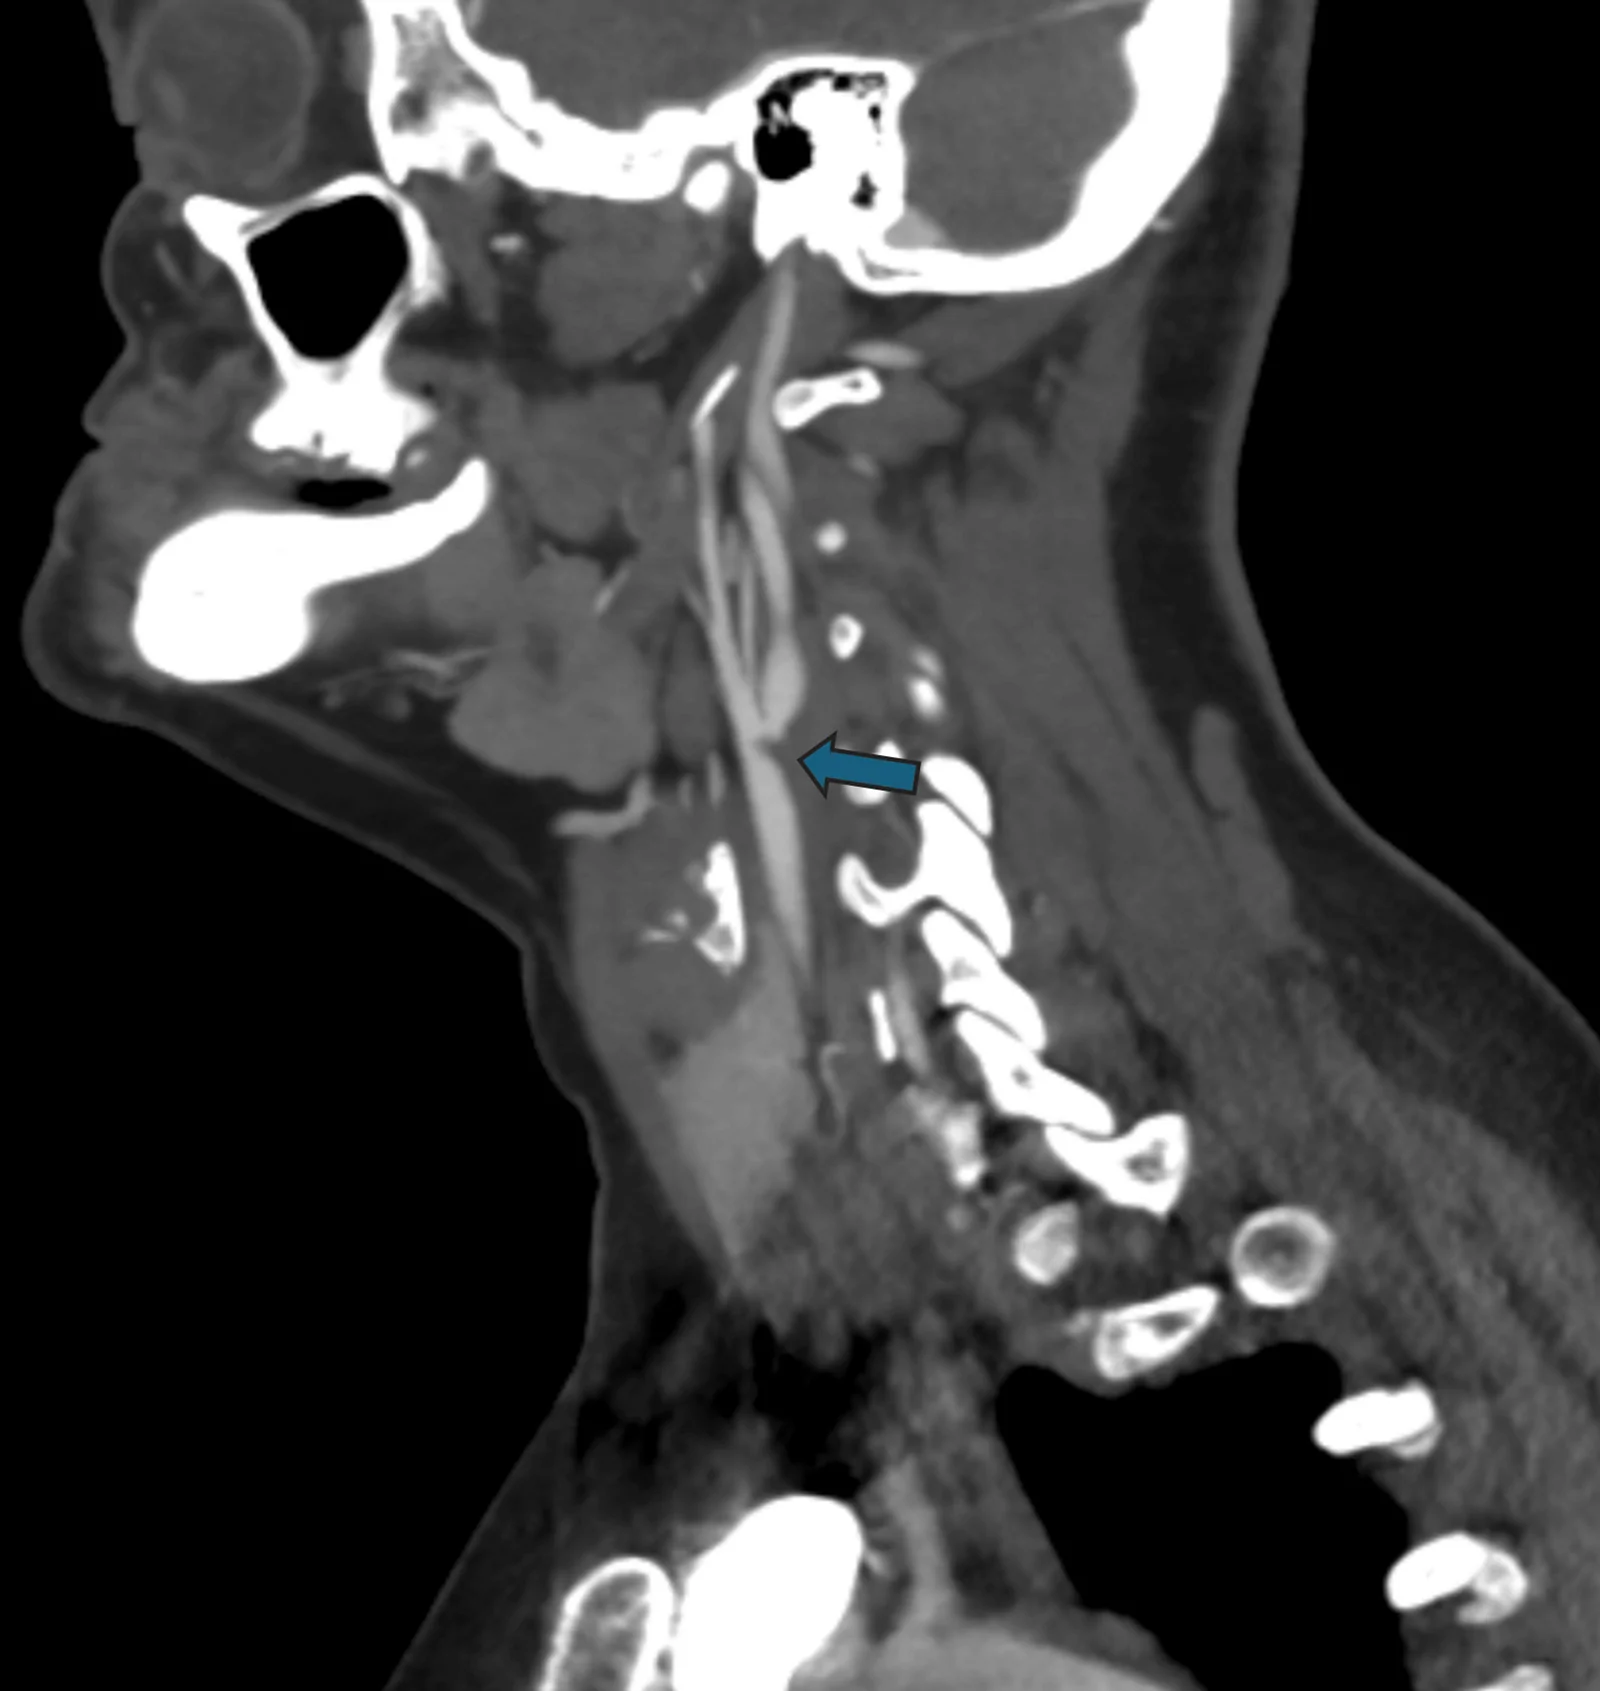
CT angiography of the neck demonstrating a smooth shelf-like hypo-density at the posterior aspect of the internal carotid artery origin (arrowed) causing a mild stenosis (North American Symptomatic Carotid Endarterectomy Trial (NASCET) 31%) with associated post-stenotic dilatation, which is in keeping with a carotid web.
